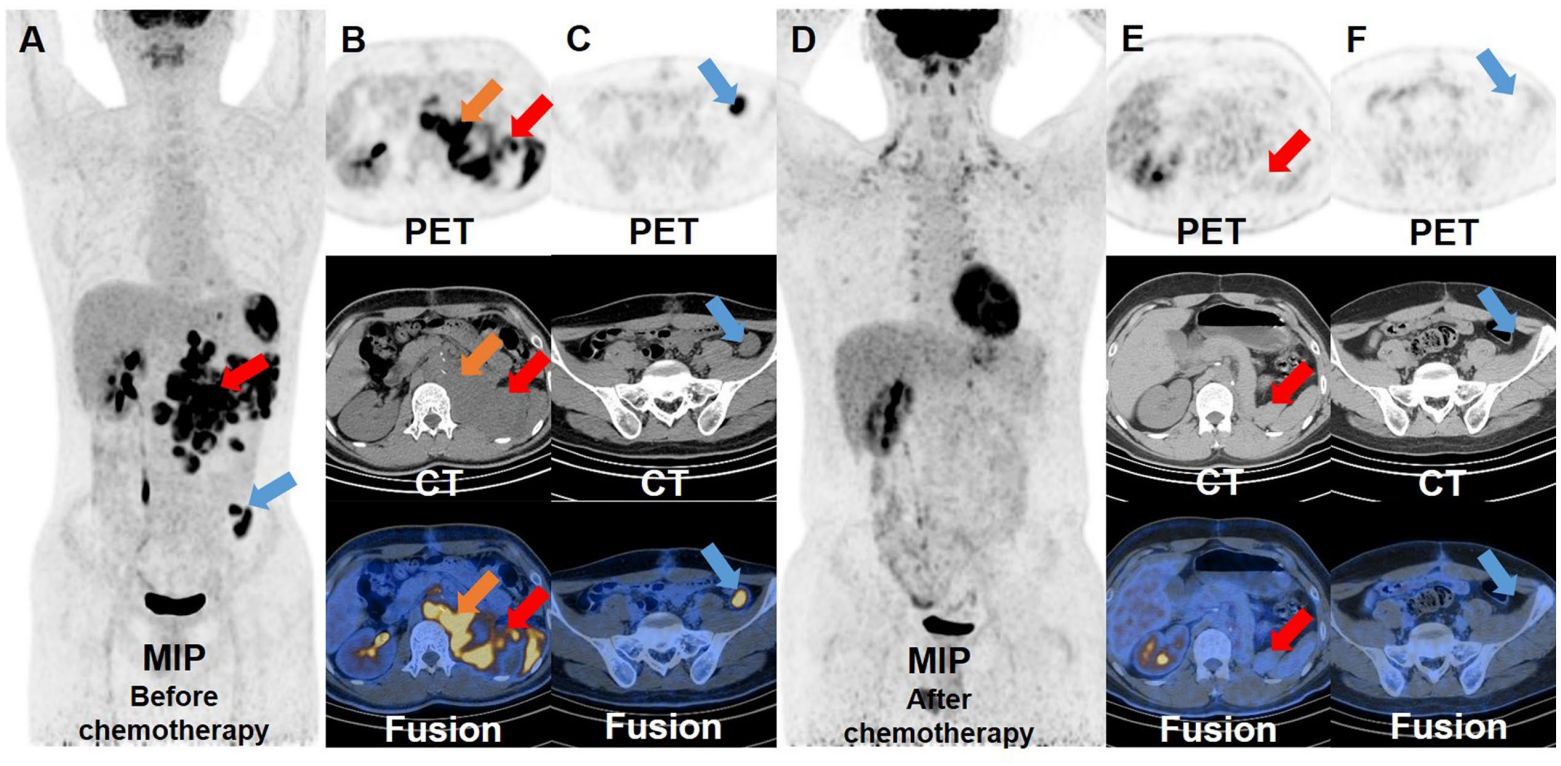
^18^F-FDG PET/CT images before and after chemotherapy. Before the patient received chemotherapy, the whole-body maximum intensity projection (A) showed multiple hypermetabolic lesions around the abdominal aorta, spleen, left psoas major, left diaphragm foot and left pelvic cavity. And the transverse images (B,C) showed that these lesions had significantly increased FDG uptake, with a SUVmax of 22.5. After the patient received a cycle of chemotherapy, the whole-body maximum intensity projection (D) and the transverse images (E,F) showed that the pre-existing lesions shrank or disappeared, and the remaining lesions displayed a marked reduction in FDG uptake compared to before.
